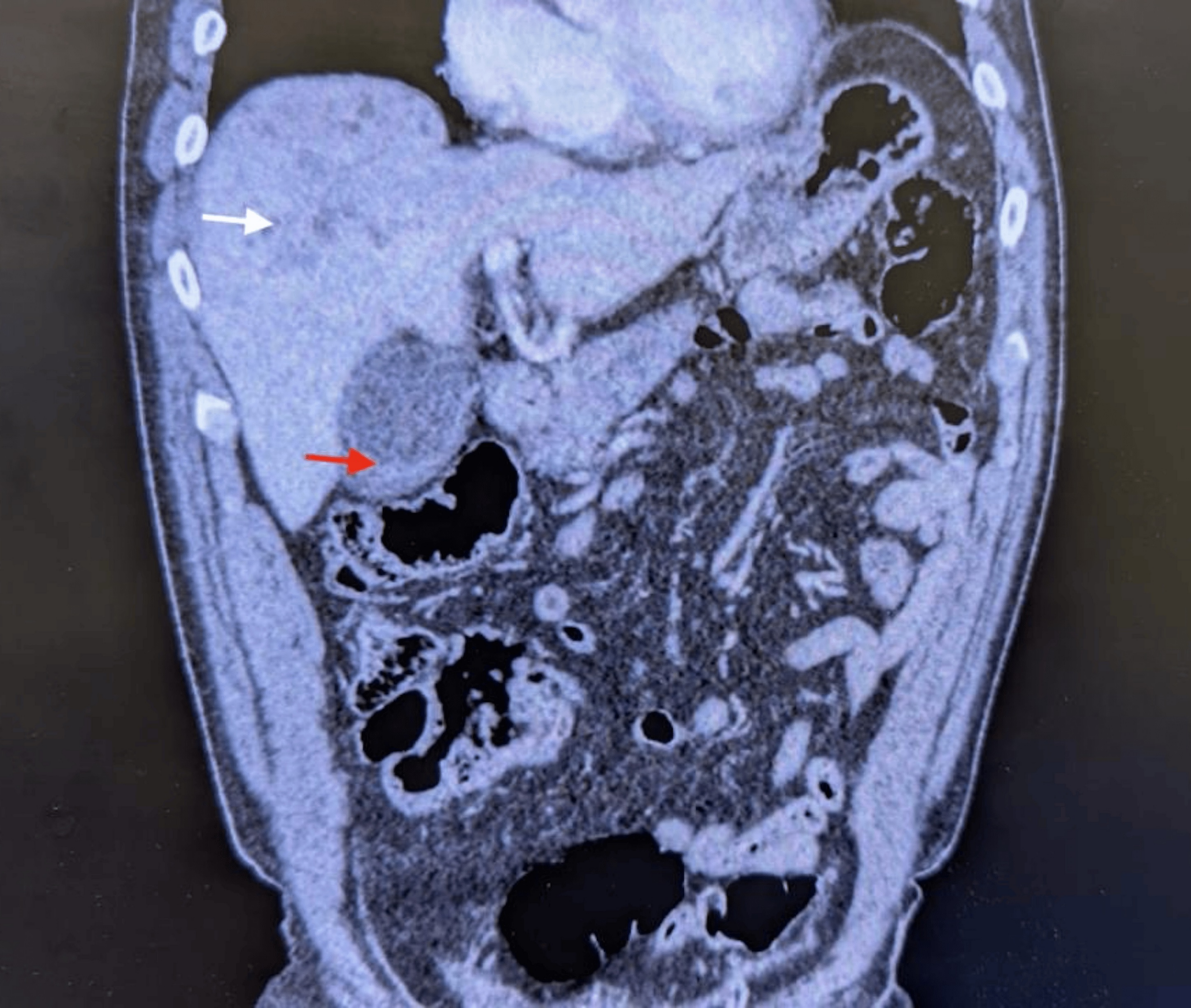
Patient's abdominal CT showing intrahepatic lesions (white arrow) and cholecystitis (red arrow).
